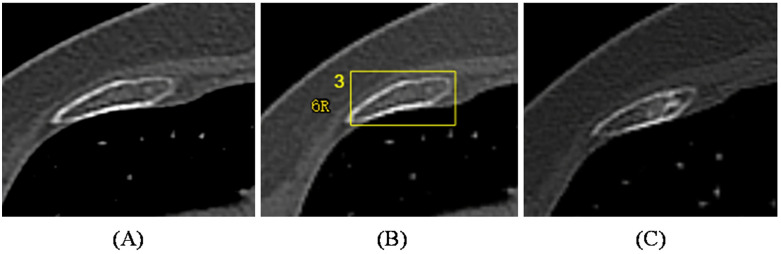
Representative case of incomplete rib fracture detection by the radiologist-CAD reading method. (A) A 63-year-old male with a car accident injury showed only a cortical fold of the bone in the right sixth anterior rib. (B) The incomplete fracture (rectangular box marked) was missed in the radiologist-only reading, whereas it was accurately diagnosed in the radiologist-CAD reading. (C) Four weeks later, a follow-up computed tomography review showed the formation of a callus at the location, confirmed as an incomplete fracture by the panel.
